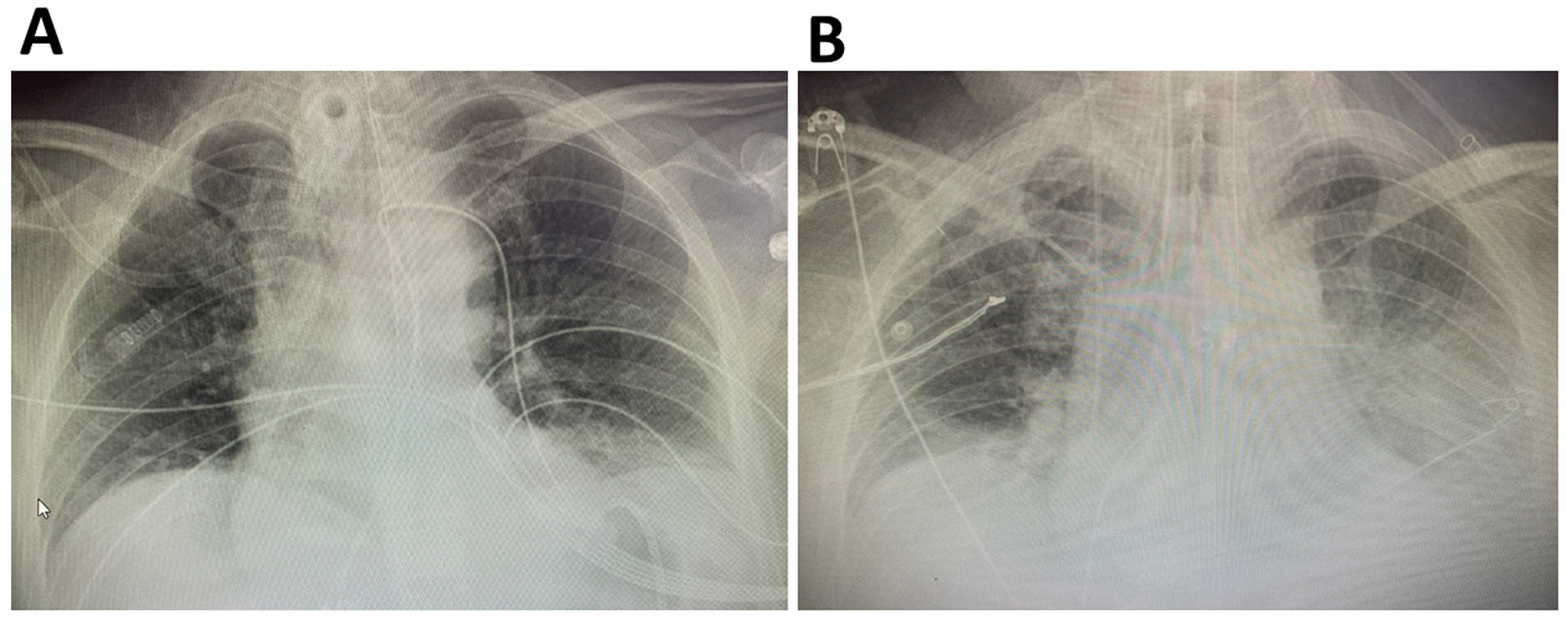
Chest X-rays before and after IPE initiation Chest X-rays demonstrating pulmonary status prior (A) and two weeks after (B) IPE initiation.
